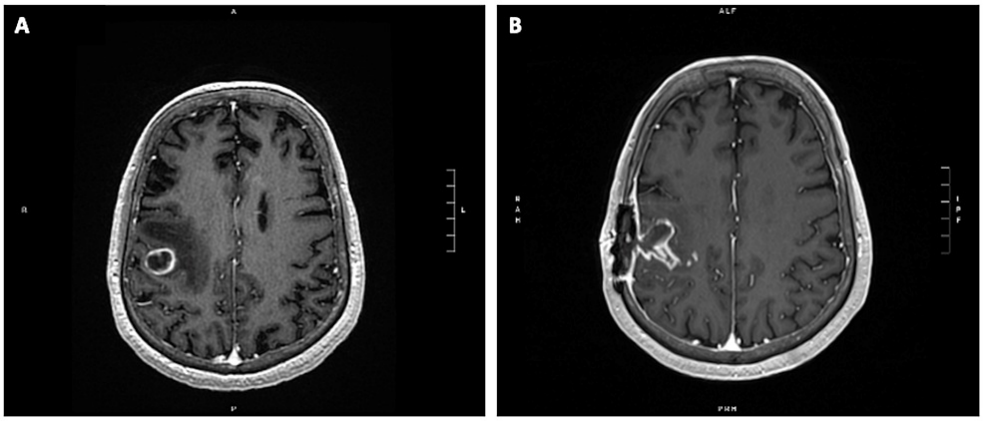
Preoperative and postoperative imaging of the posterior right frontal lobe brain metastasis (A) Preoperative T1 MRI with contrast showed the 1.8 cm intact brain metastasis with surrounding edema extending from precentral gyrus to the frontal subcortical white matter with mild mass effect. (B) A postoperative T1 MRI demonstrated postsurgical changes. This postoperative MRI was fused with a CT scan for treatment planning.
